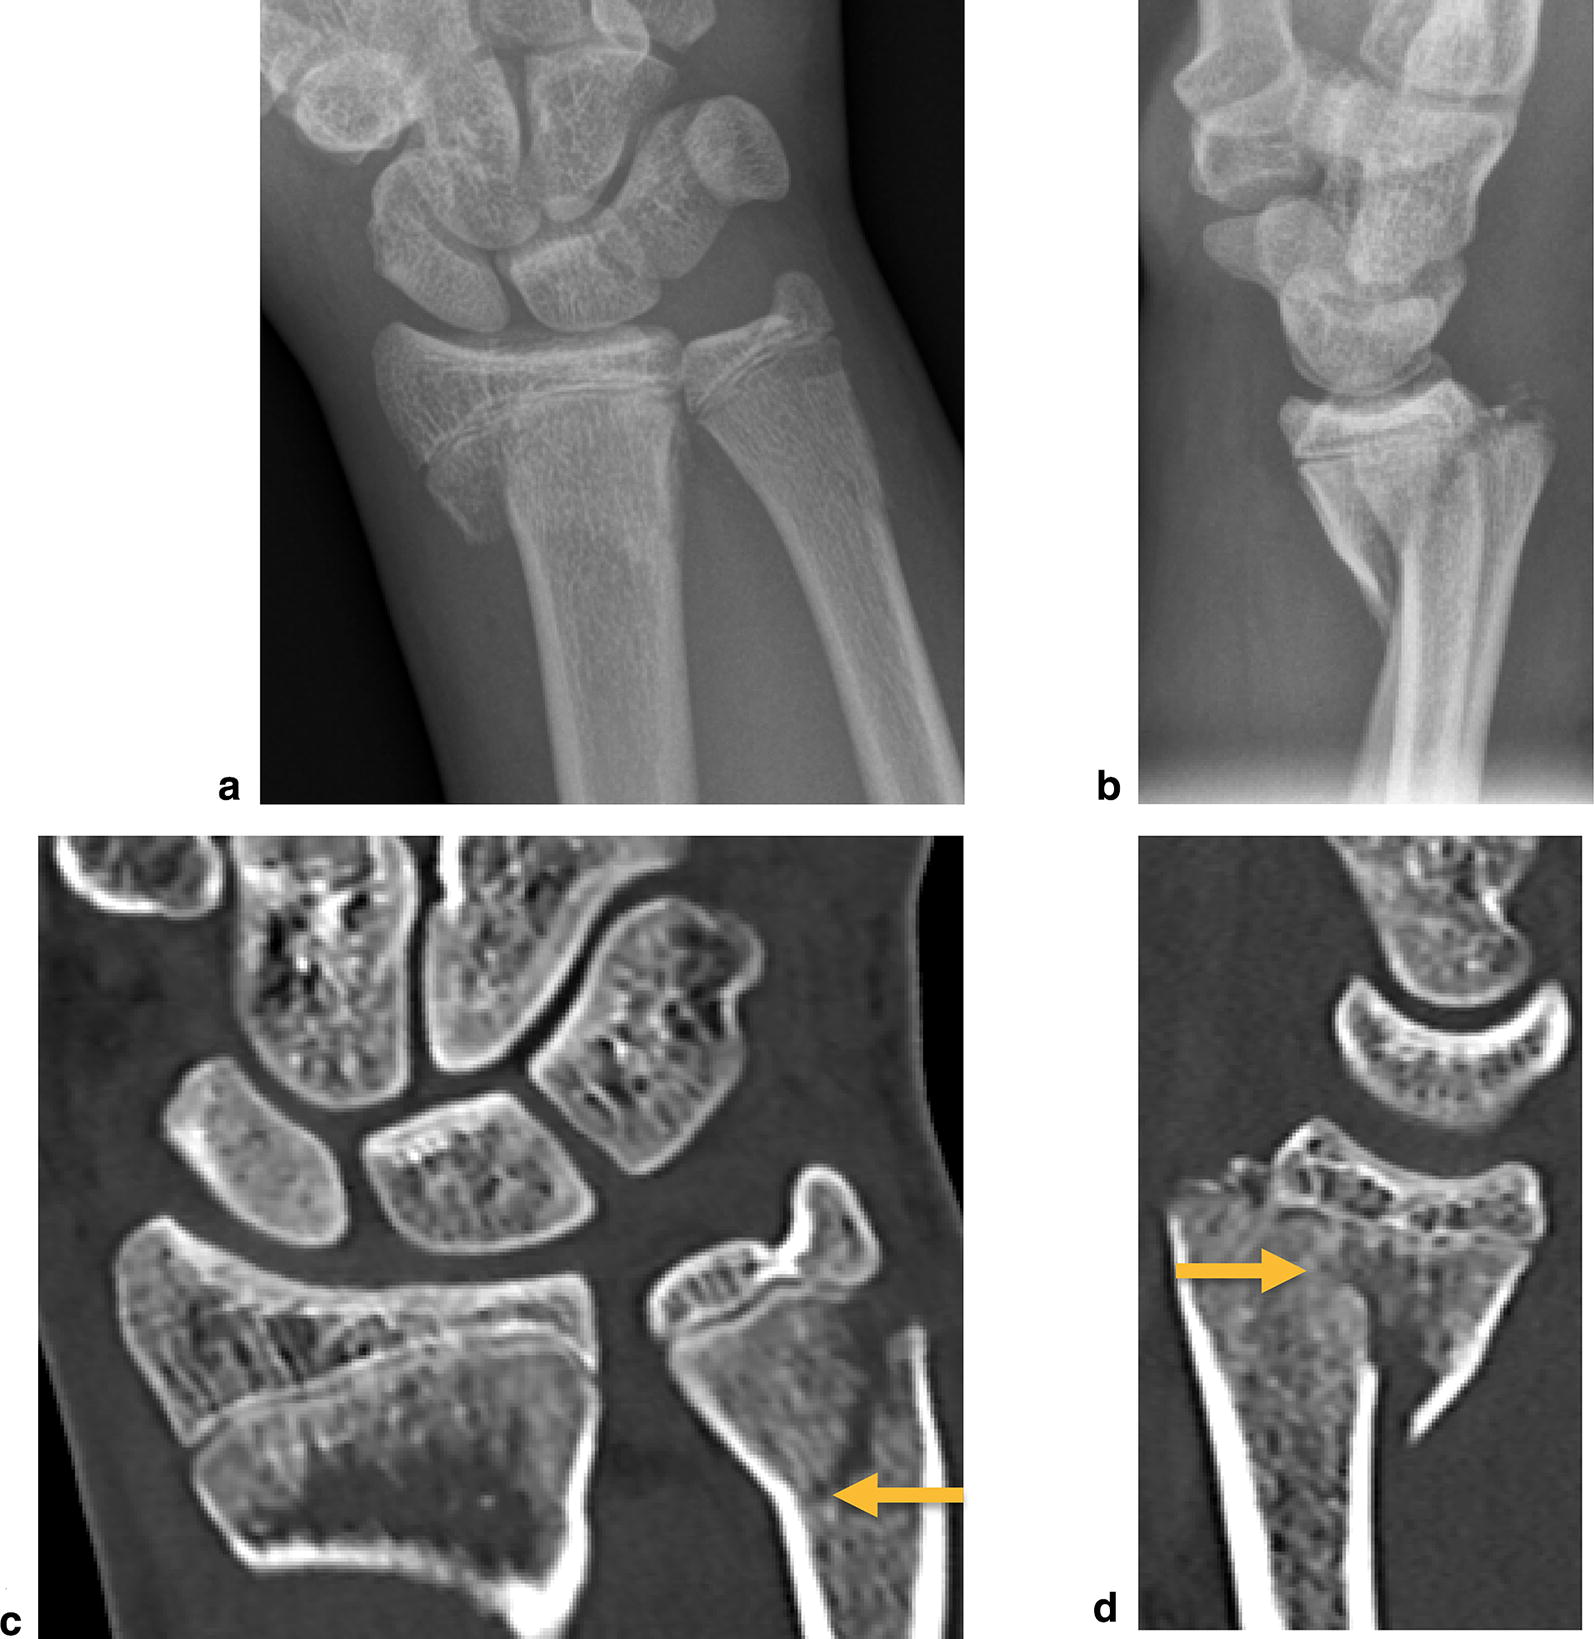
Diagnostic imaging case 1. Radiograph (a antero-posterior view; b lateral view) and CT scan (c coronal view, arrow indicating transitional fracture of the distal ulna; d sagittal view, arrow indicating transitional fracture of the distal radius) of the right wrist, showing a transitional both of the distal radius and the distal ulna
